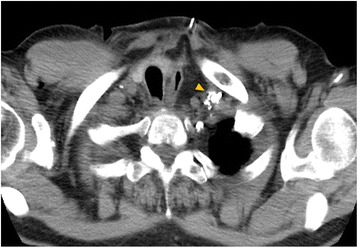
Coronal sections by CT obtained a day after lipiodol lymphangiography show the accumulation of lipiodol at the left venous angle (*arrowhead*)
